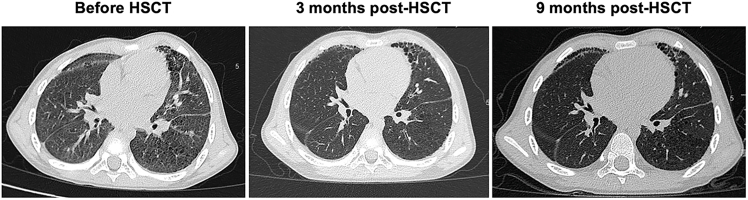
Serial chest CT scans before and after HSCT High-resolution chest CT images obtained before HSCT, 3 and 9 months after HSCT, showing bilateral ground-glass opacities, panlobular emphysema, and fibrotic changes with similar extent and distribution at each time point.
